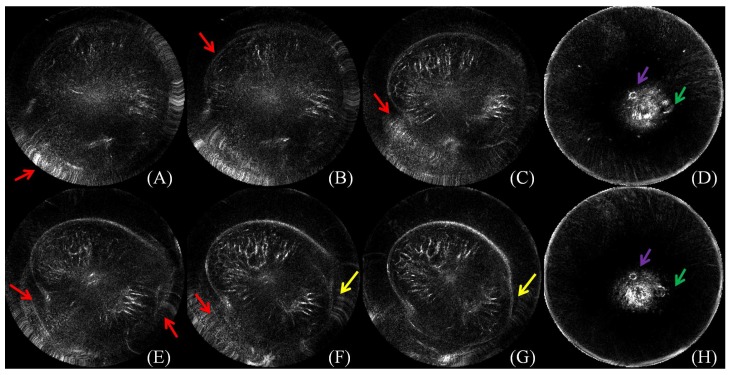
(A–H) The challenges of breast segmentation in B-mode ultrasound tomography (UST) images. Red arrows indicate the major artifacts due to the limited space between the breast boundary and the ring transducer; yellow arrows indicate unnatural distortion of the breast shapes; purple arrows indicate the second kind of circle-like artifacts; and green arrows indicate the real nipple regions. Annoying stripes (A–C, E–G) and unnatural breast shapes (C, E–G) are observed. Moreover, artifacts with circle-like shapes (indicated by purple arrows) look like nipples (indicated by green arrows) (D, H). The figure can be enlarged to view the details.
